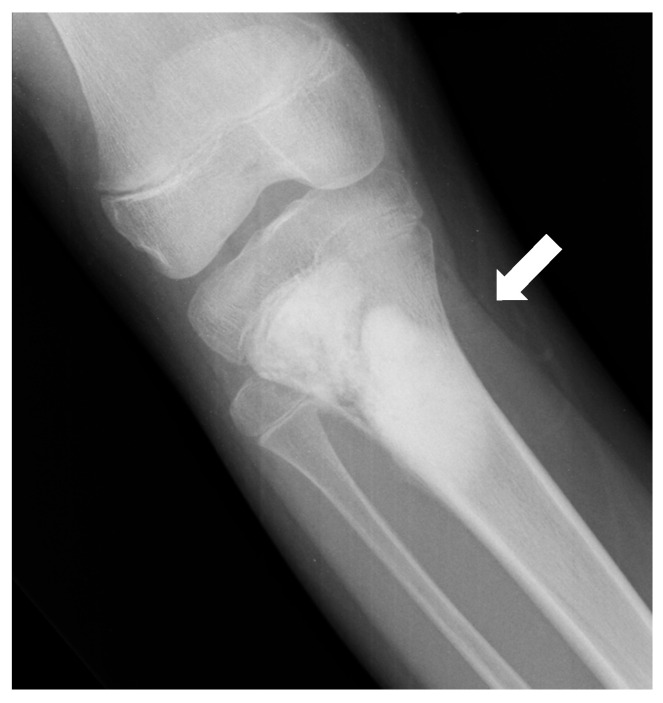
Pure bone-forming osteosarcoma. Conventional radiograph of the right knee (AP projection) of a 10-year-old female diagnosed with conventional (osteoblastic) osteosarcoma of the proximal tibia. A purely sclerotic pattern is observed at the proximal metaphysis of the tibia (arrow).
